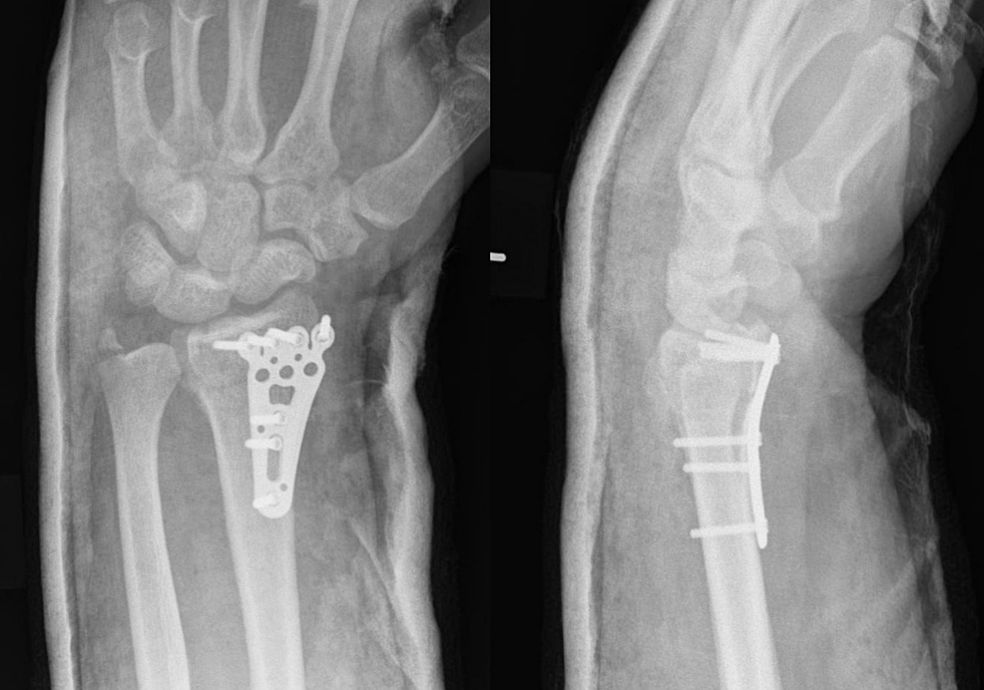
Postoperative radiographs showing a misplaced screw radial to the radial styloid and locking screws too short for osteoporotic bone
